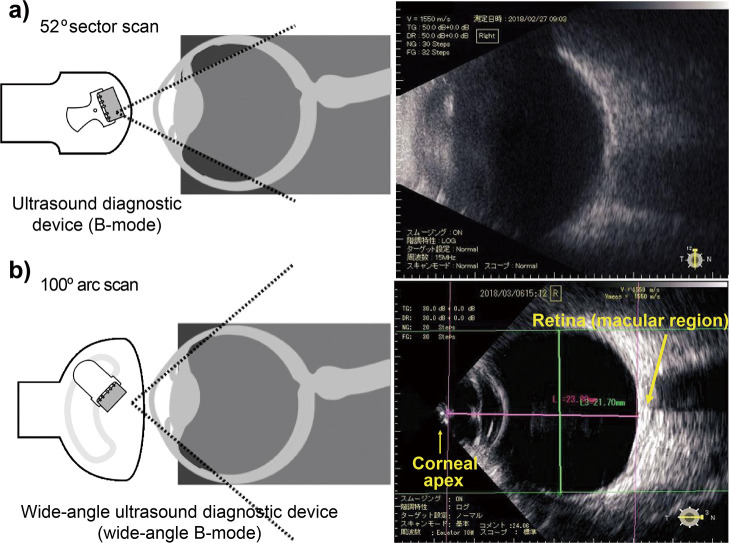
Images obtained using (a) a conventional ultrasound diagnostic B-mode device and (b) a wide-angle ultrasound diagnostic B-mode device The imaging range is wider with the wide-angle ultrasound diagnostic B-mode device than with the conventional B-mode device. The distance from the corneal apex to the retinal macular region, indicated by an arrow, was measured as AL; the maximum diameters across the peripheral retina of horizontal and vertical lines that intersected with the AL were defined as the HD and VD, respectively. AL, axial length; HD, horizontal distance; VD, vertical distance (Extracted from Figure 2 from Atchison DA, Jones CE, Schmid KL, Pritchard N, Pope JM, Strugnell WE, et al. Eye shape in emmetropia and myopia. Invest Ophthalmol Vis Sci. 2004;45:3380–6 with permission).
